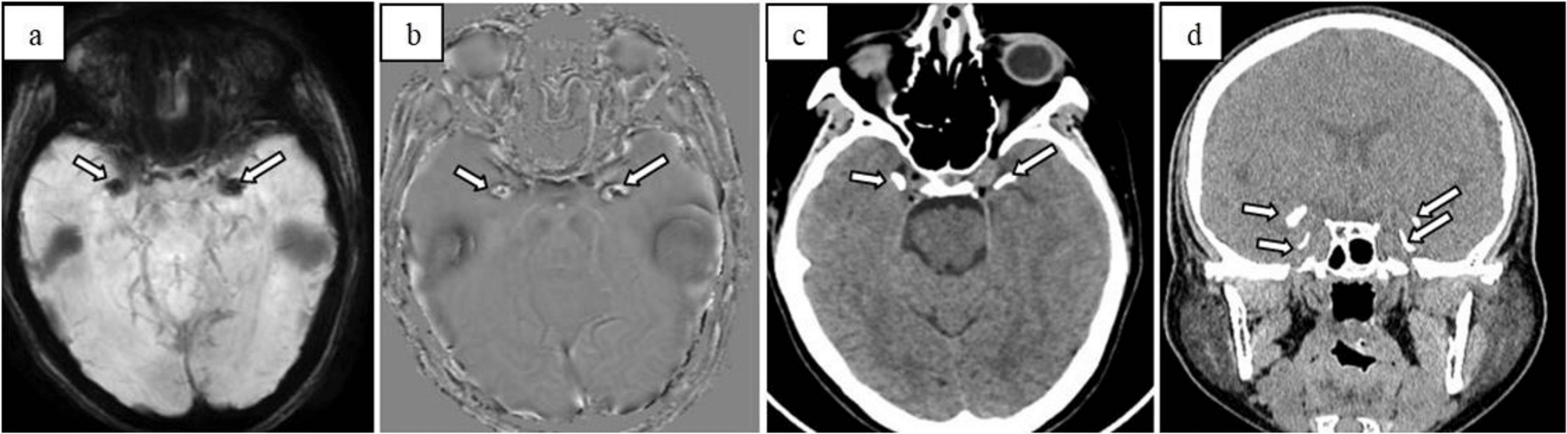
(a, b) susceptibility image showing blooming in the bilateral symmetrical medial temporal lobes with a corresponding bright signal on phase image (arrows), suggestive of calcifications. (c) Axial and (d) coronal CT images showing bilateral symmetrical medial temporal lobe calcifications (arrows). Note that the calcifications are bean and horn-shaped.
